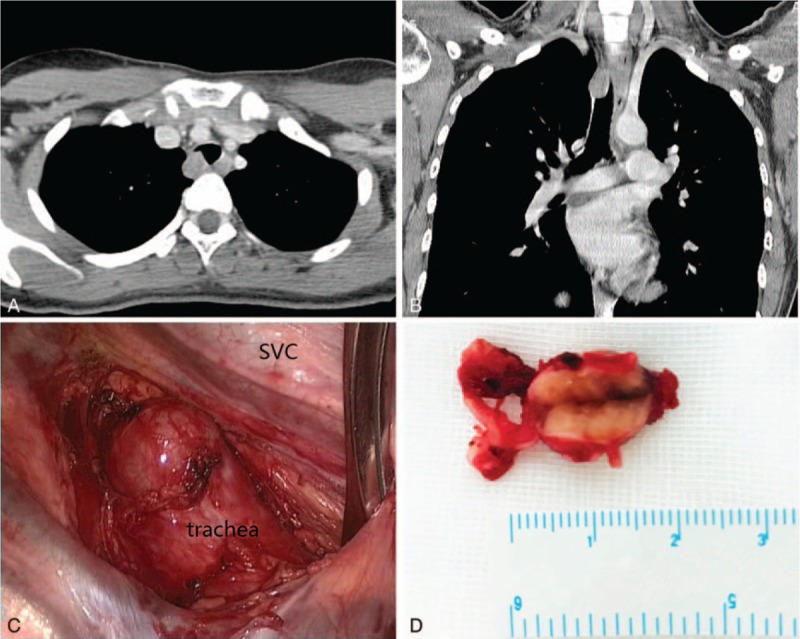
Intratracheal schwannoma on CT scan and intraoperative findings. A and B: Enhanced and 3-dimensional reconstructive CT indicated the tumor with an irregular shape, obscure boundary and moderate enhancement, and protruded into the right thoracic cavity obviously. C and D: Intraoperative findings: The tumor had an integrated capsule with several small vessels (arrow). The cut surface revealed a pale-yellow appearance, partially intermingled with brown. SVC = superior vena cava.
